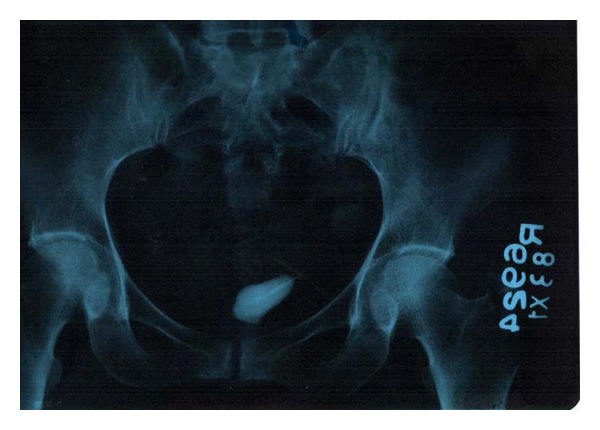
Plain X-ray showing the calculus and the horizontal limb of the device attached to it.
